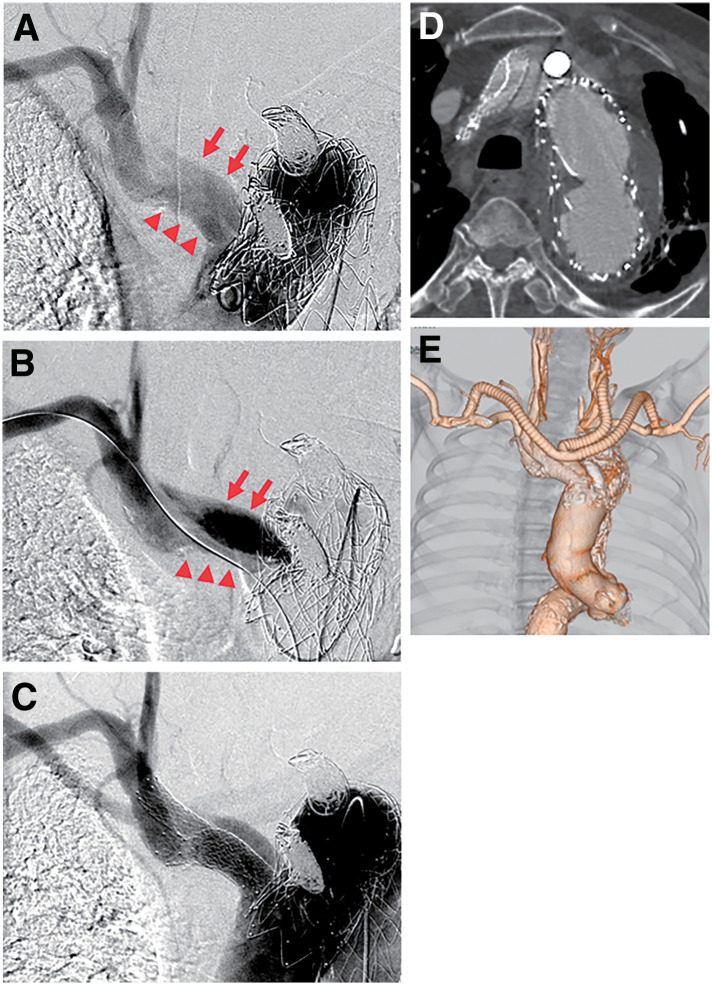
Angiographic findings during re-intervention and postoperative CECT. (A) Aortography on the day of symptom onset revealing BCA dissection with visualization of the false lumen (arrows) and preserved true lumen flow (arrowheads). (B) Selective angiography performed the following day showing a progression of false lumen expansion (arrows) and worsening true lumen compression (arrowheads). (C) Aortography after kissing stent placement from the BCA to the right subclavian and right common carotid arteries, revealing a restoration of true lumen flow without extension into the aorta. (D) Axial CECT prior to discharge showing adequate stent expansion. (E) Volume-rendering image confirming stent patency and bypass graft enhancement. CECT: contrast-enhanced computed tomography; BCA: brachiocephalic artery
